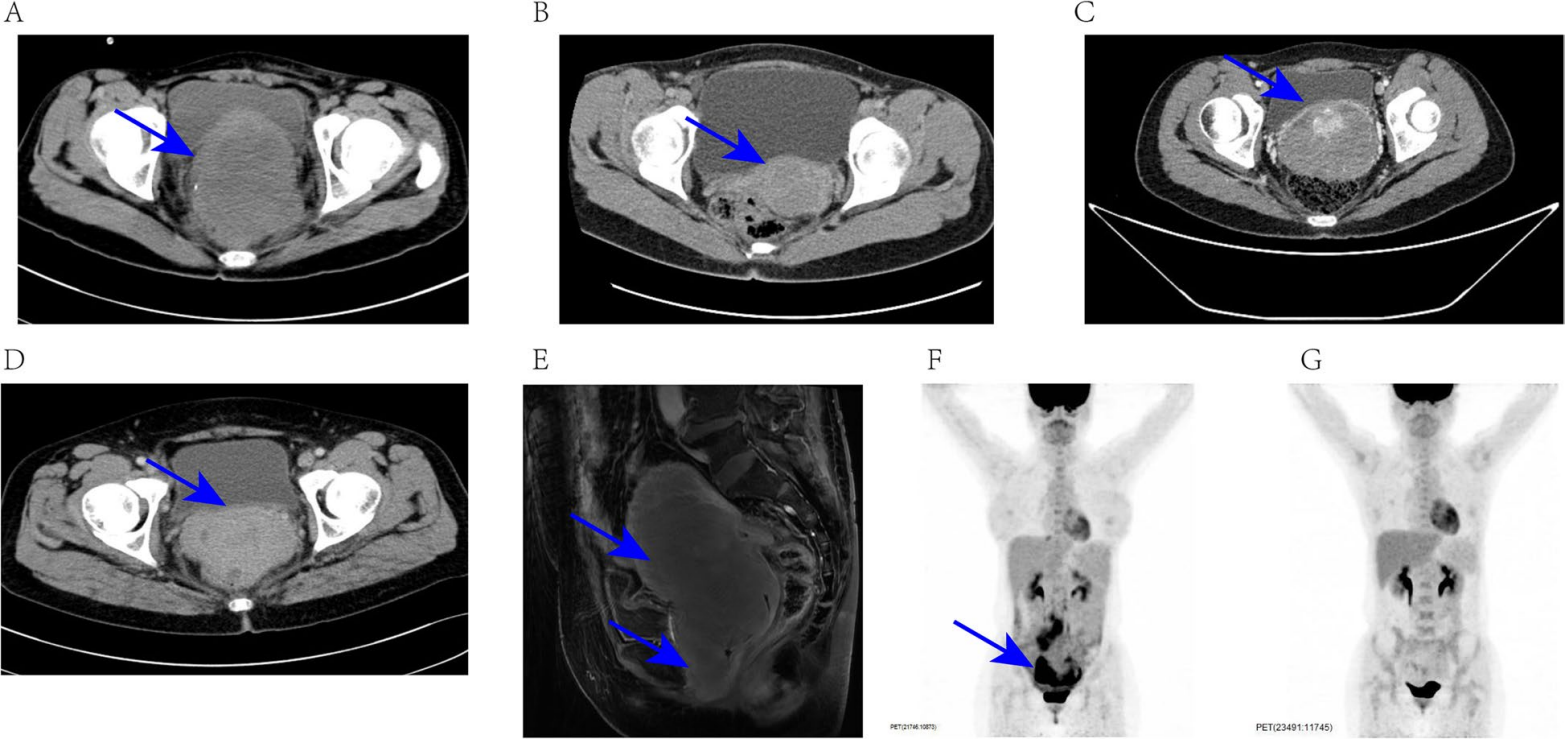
CT/MRI/PET-CT images of patients. A CT image of pelvic mess from patient 1(tumor size = 10 cm); B CT image of cervical mess from patient 3 (tumor size = 4 cm); C CT image of cervical mess from patient 7 (tumor size = 8.4 cm); D CT image of cervical mess from patient 8 (tumor size = 5.7 cm); E MRI image of cervical and vaginal mess from patient 4 (tumor size = 5 cm, 5 cm); F (before treatment) and G (after treatment) are PET-CT images of body from patients 6
